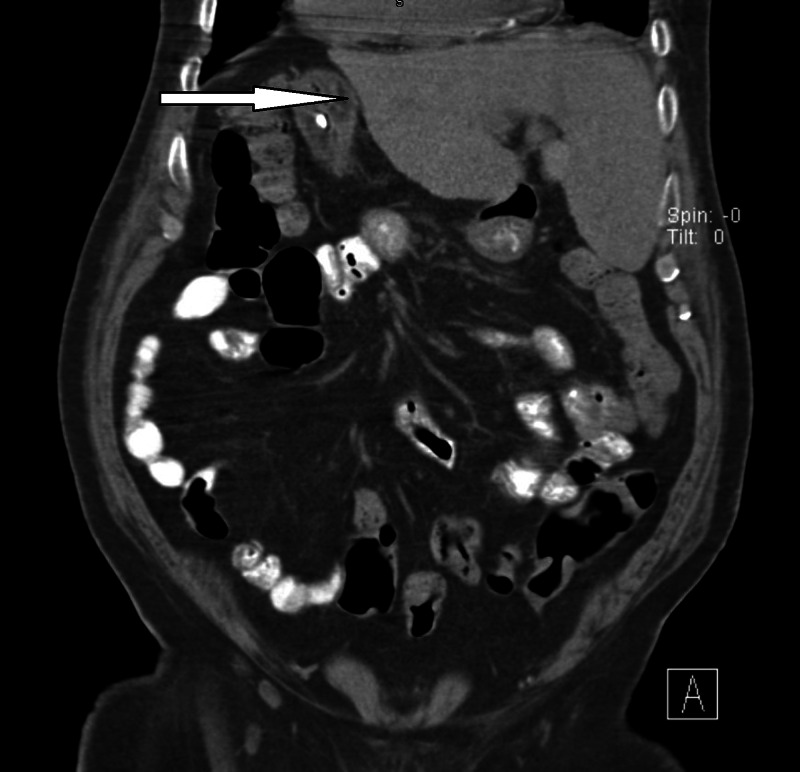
Coronal CT scan abdomen of a patient with SIT Arrow pointed to the liver, located on the left side in a patient with SIT. SIT, Situs inversus totalis.
